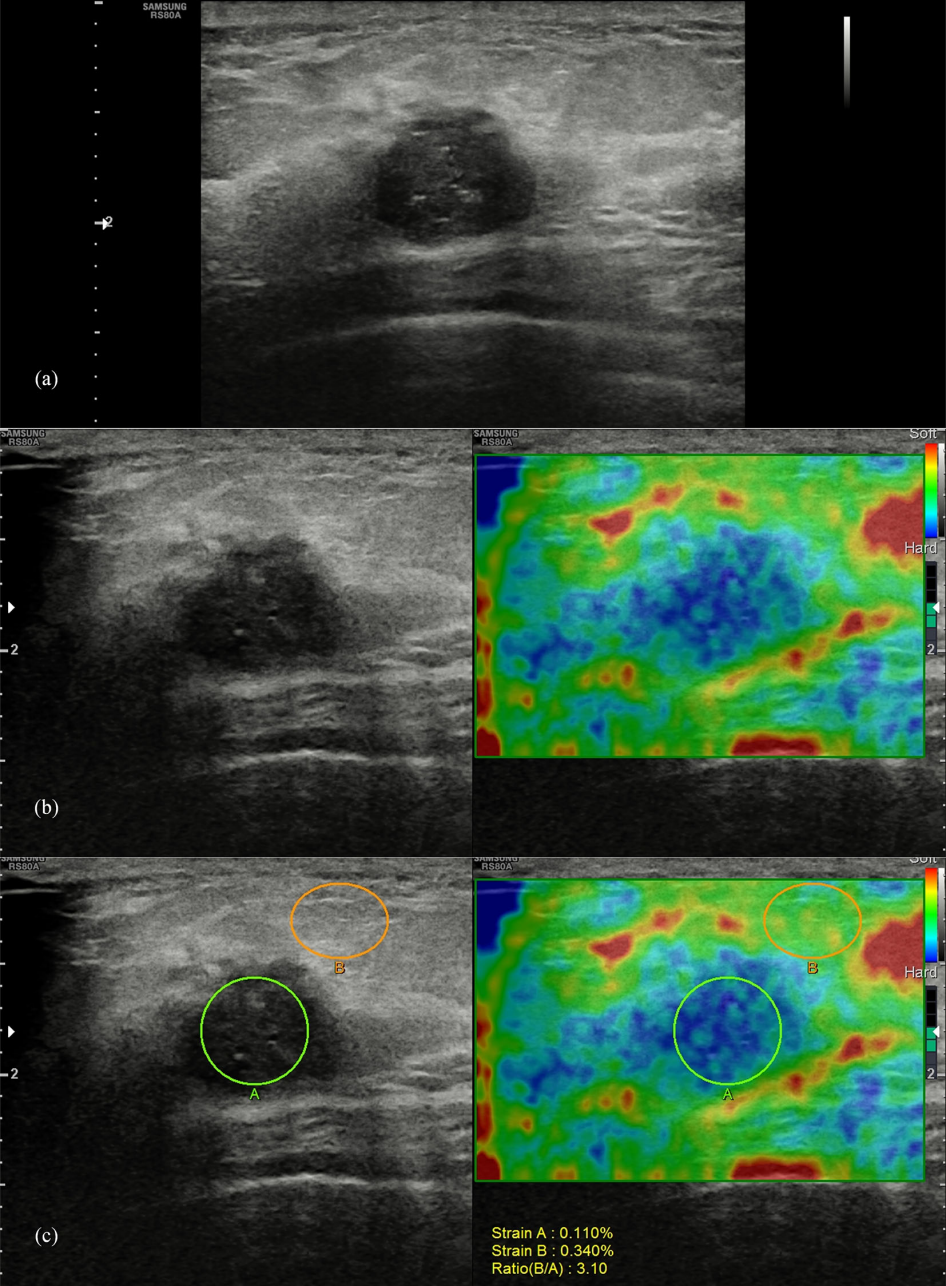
Pathologically confirmed breast invasive ductal carcinoma in a 44-year-old female patient. Ultrasound images of the long-axis section of the breast mass were evaluated as BI-RADS 4c (A), with an elasticity score of 4 (B), and a strain ratio of 3.1 (C).
